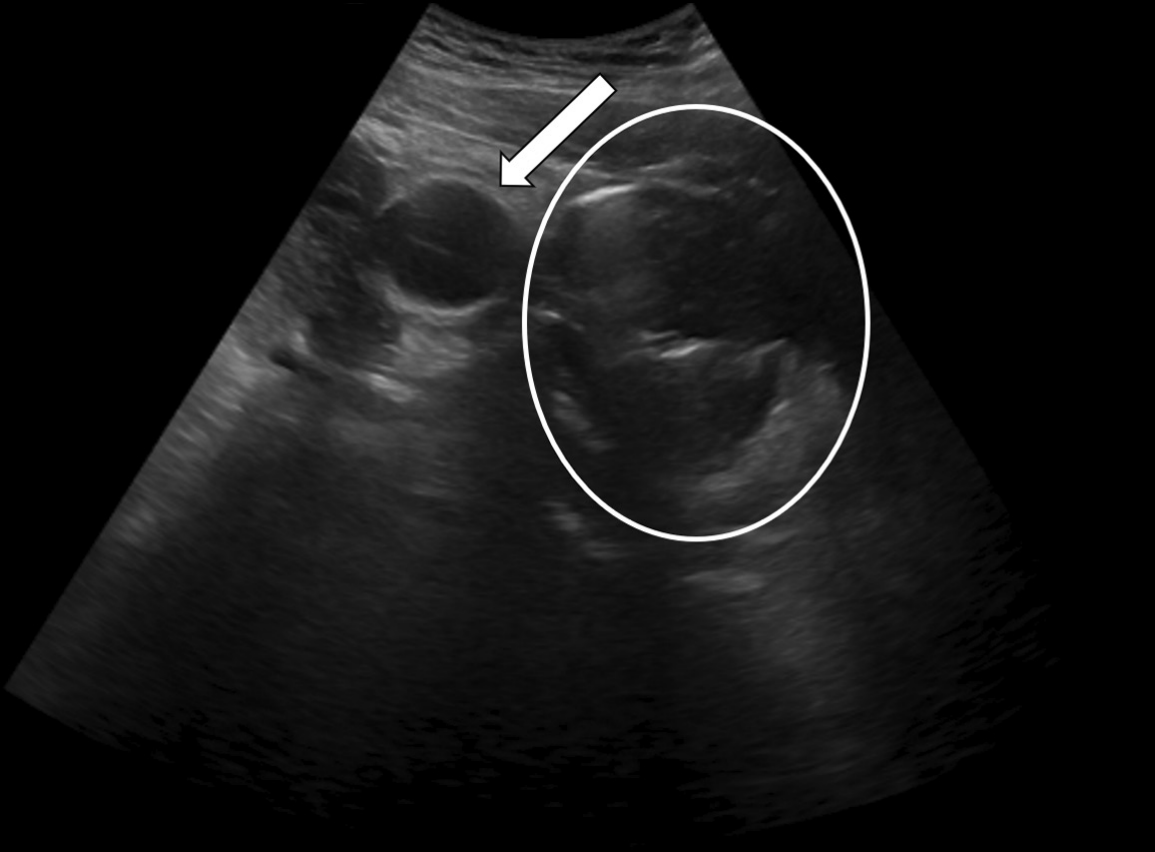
Ultrasonography image showing foley's bulb (white arrow) lying outside urinary bladder (white circle).
